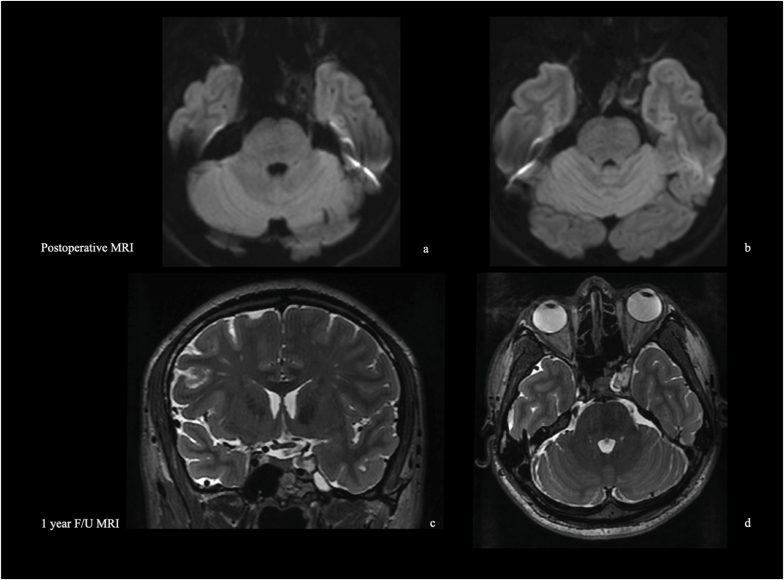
Postoperative MRI demonstrating total cyst removal with subtotal capsule excision in the superoposteromedial portion of the lateral wall of the cavernous sinus (LWCS). The immediate postoperative DWI MRI (a, b) shows no diffusion restriction except for a minimal focal signal corresponding to the capsule remnant (b). At the 1-year follow-up, T2-weighted MRI in coronal and axial planes (c, d) reveals decompression of the cavernous sinus and a signal intensity isointense to CSF, indicating absence of recurrence.
